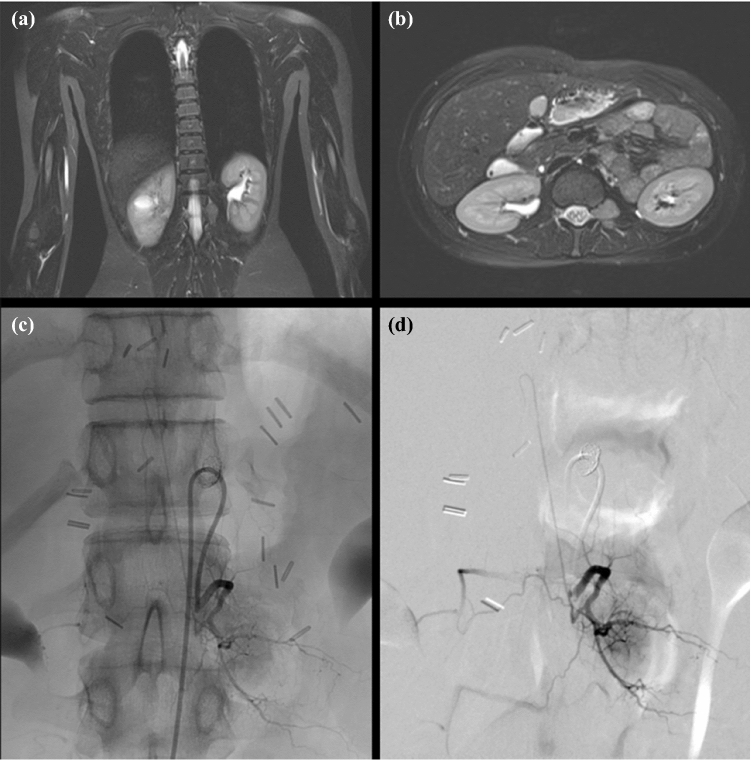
Left-sided, paravertebral local relapse of hepatocellular carcinoma in an 18-year-old girl (patient 4). (a) Coronar and (b) axial MR images. The tumor is located from T12 to L2. (c) Coronar and (d) sagittal images of selective spinal angiography. The origin of the artery of Adamkiewicz is at level L1 on the left. Surgery was abandoned, and proton beam therapy was performed.
